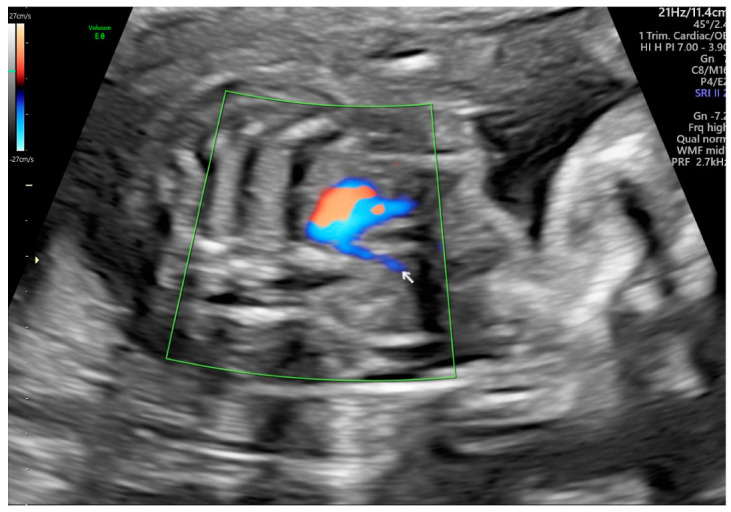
Color Doppler ultrasound images of a fetal aberrant right subclavian artery at 21 weeks of gestation. The arrow point to the site of ARSA.
